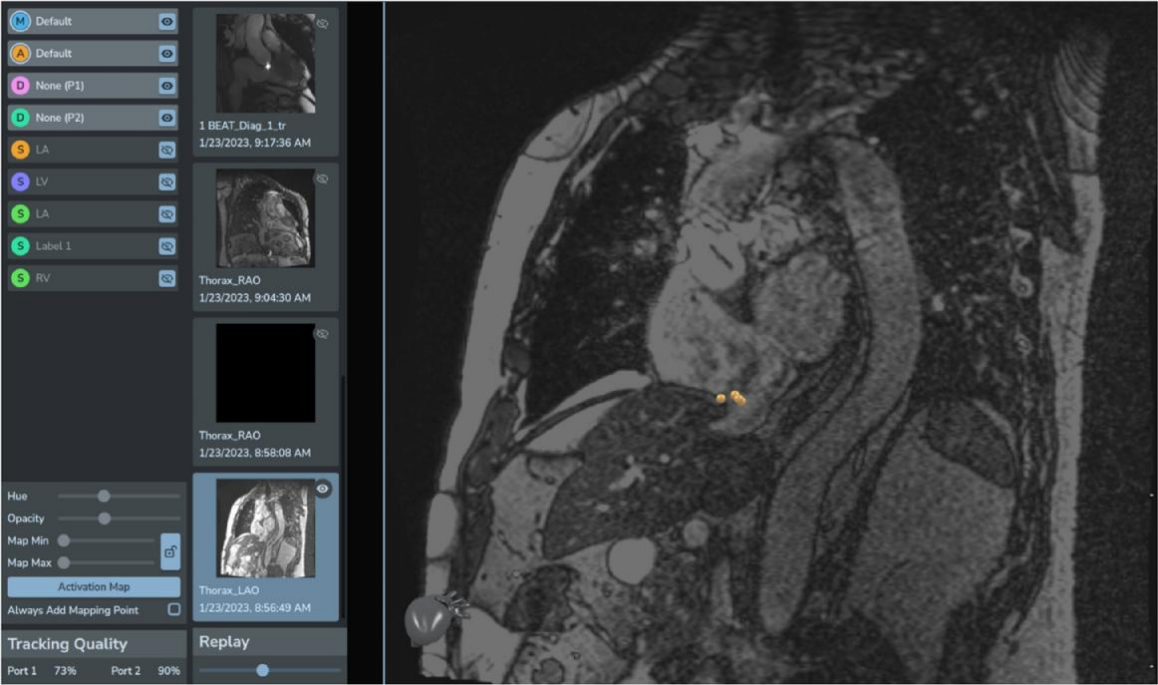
Example of the display of ablation points directly on real-time MRI images without the superimposed 3D shell. Three different ablation points are correctly localized on the CTI, confirming the good orientation of the 3D shell and thus its reliability in guiding the procedure. 3D, three-dimensional; CTI, cavotricuspid isthmus.
